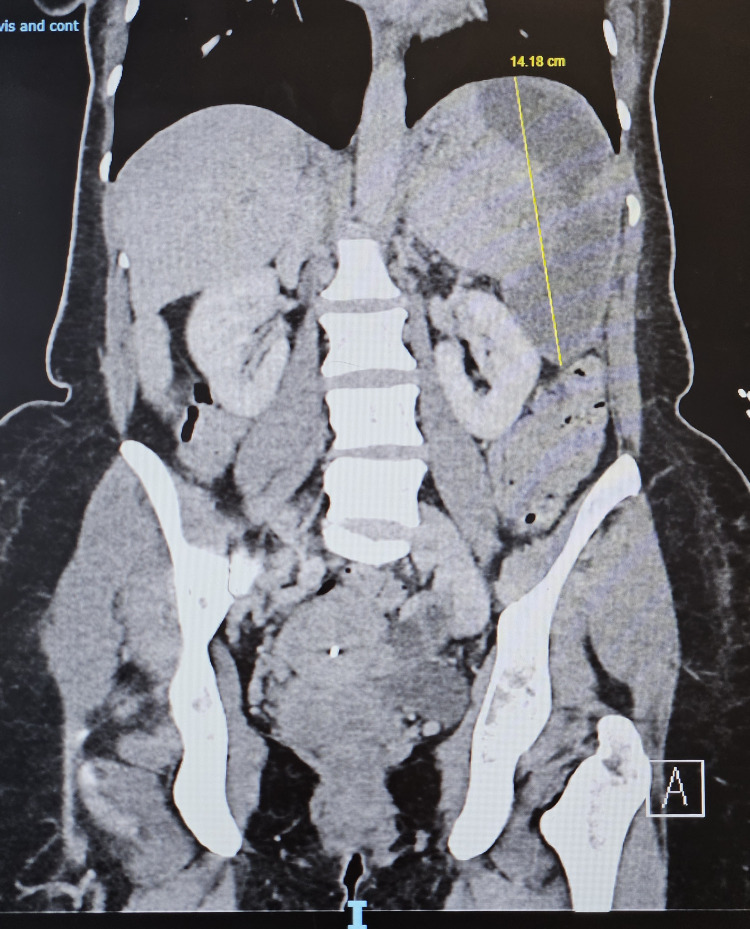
A 14 cm splenomegaly visible on CT abdomen and pelvis
